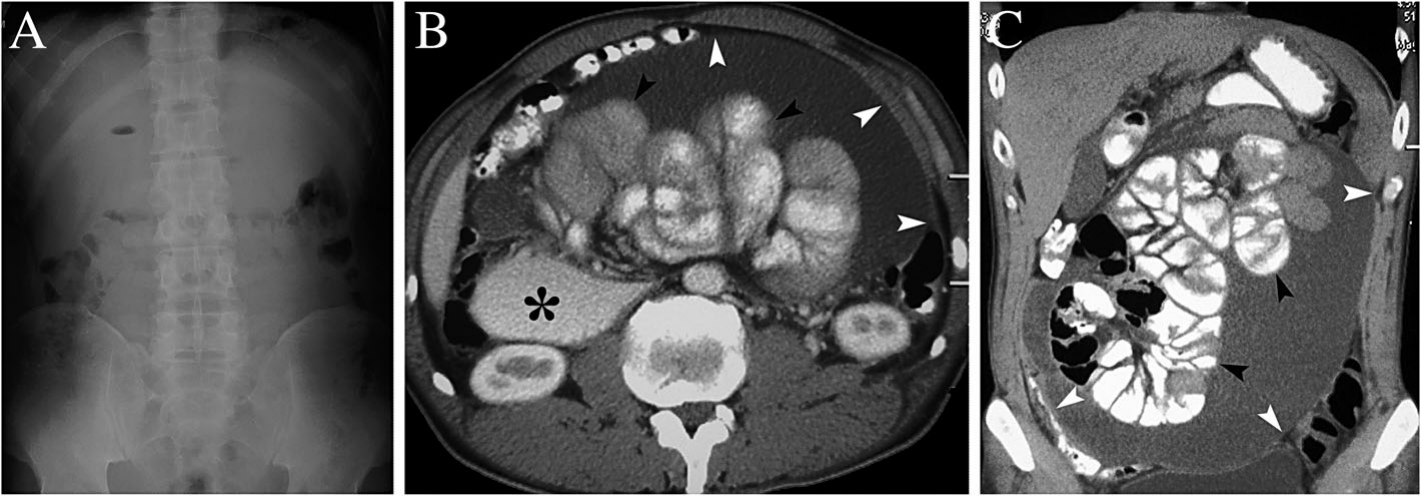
a: Plain upright abdominal X-ray showed some air in the colon without presence of multiple air fluid levels in the loops or free gas under the diaphragm; b, c: Contrast-enhanced computed tomogram scan revealed dilatation of the duodenum loops (*) and congregated small gut loops (black arrowhead) trapped in the massive ascites surrounded by a membrane (white arrowhead)
